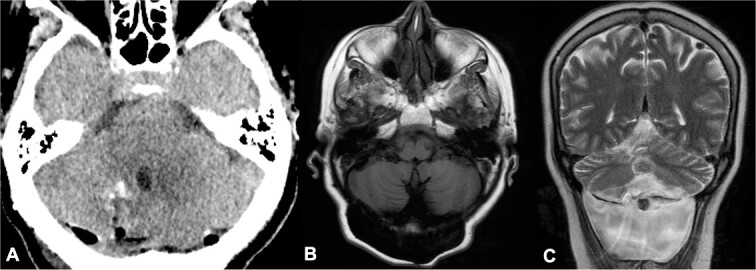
Postoperative head CT and 2-year follow-up brain MRI. (A) Axial view of head CT demonstrated complete resection of cysts; (B–C) 2-year follow-up brain MRI showed no recurrence of parasitic cysts in T1 axial and T2 coronal sequences and a cerebrospinal fluid fistula.
